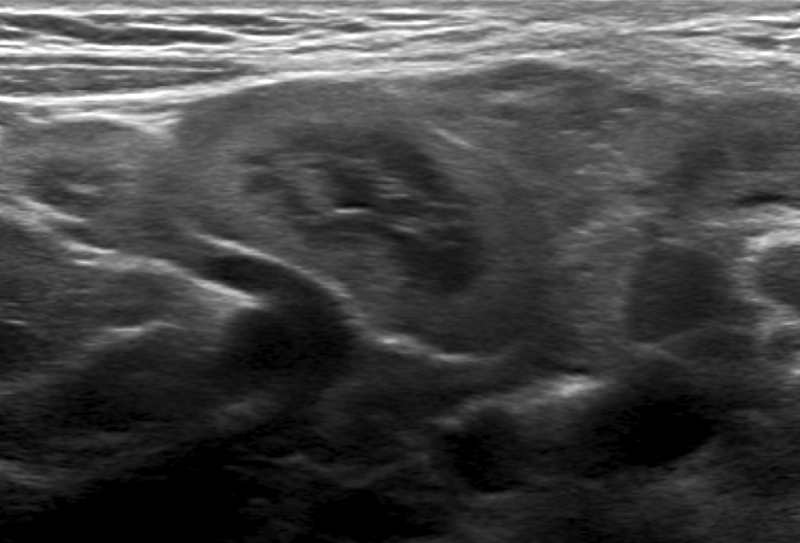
Greyscale ultrasound image demonstrating the distinctive hyperechogenic cortex and hypoechogenic medulla.
